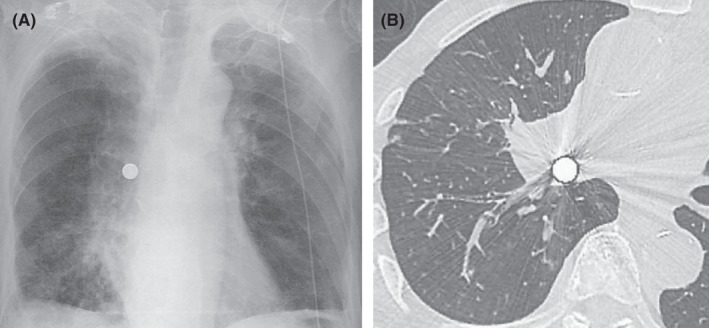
Chest radiograph (A) and CT scan (B) show a round high‐density shadow nearly 1cm in diameter. The right inferior lobar bronchus seems to be obstructed by a metallic round substance.
